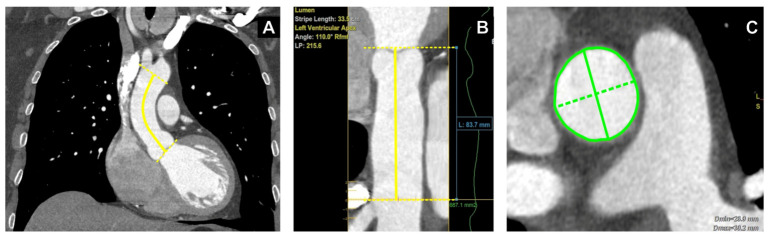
Computed tomography angiography (CTA) based morphometric assessment of the ascending aorta. (A) Coronal CTA overview of the thoracic aorta showing the analyzed segment of the ascending aorta. The solid yellow curved line indicates the aortic centerline while the dashed yellow transverse lines at the ends of the solid line indicate the proximal and distal landmarks used for ascending aortic length assessment. Ascending aortic length was measured along the centerline from the aortic annulus to the origin of the innominate artery. (B) Reconstructed view of the assessed segment showing centerline-based measurement of ascending aortic length. The solid yellow vertical line indicates the measured ascending aortic length, and the yellow dashed transverse lines indicate the reference levels defining the measured segment. (C) Cross-sectional CTA image at the level of the maximal ascending aortic dimension. The solid green line represents the maximal diameter, and the green dashed line represents the perpendicular short-axis diameter. The maximal diameter, measured as the distance between two oppositely positioned points along the circumference, was recorded for analysis.
